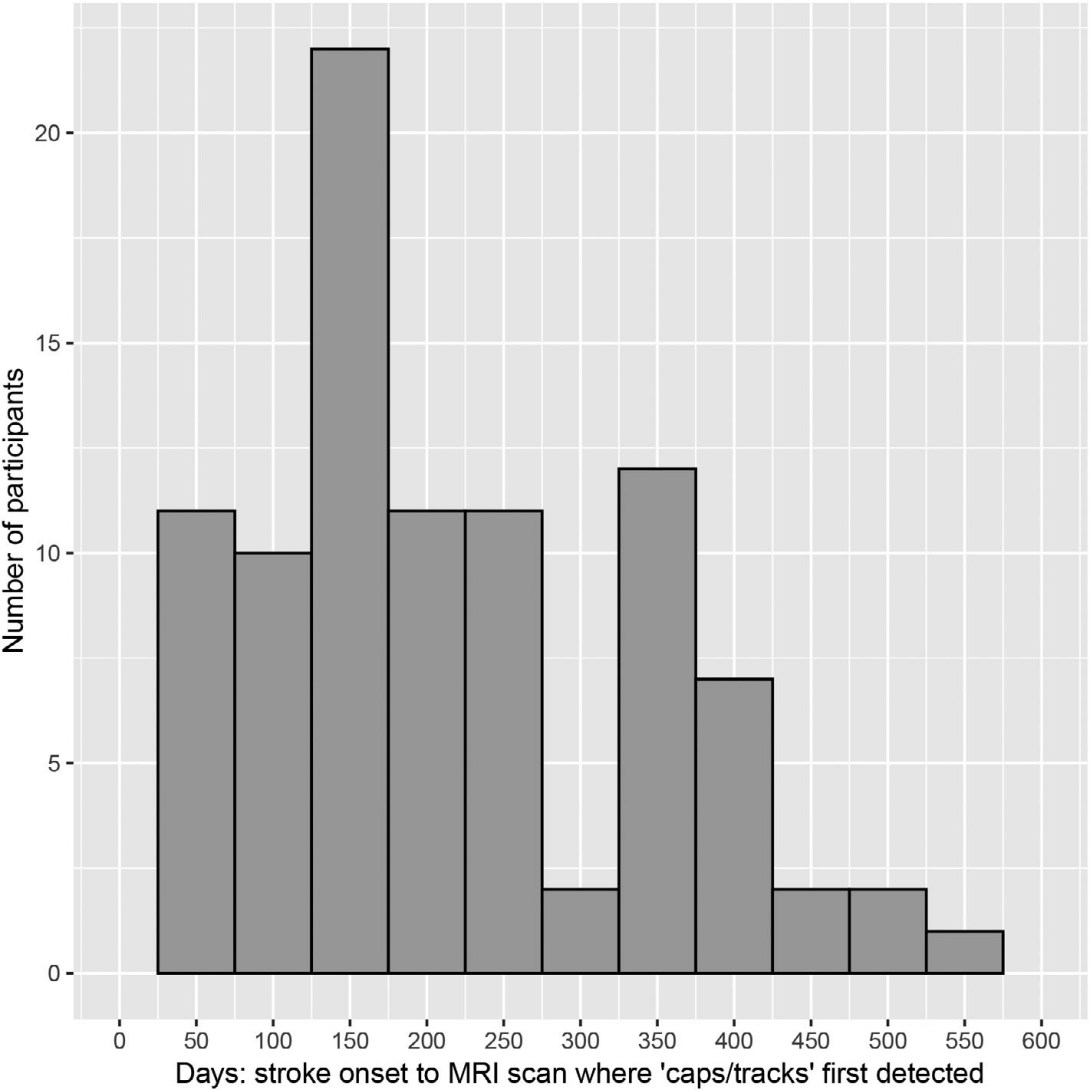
Distribution timeline of first detection of ‘caps/tracks’ on MRI across all visits.
